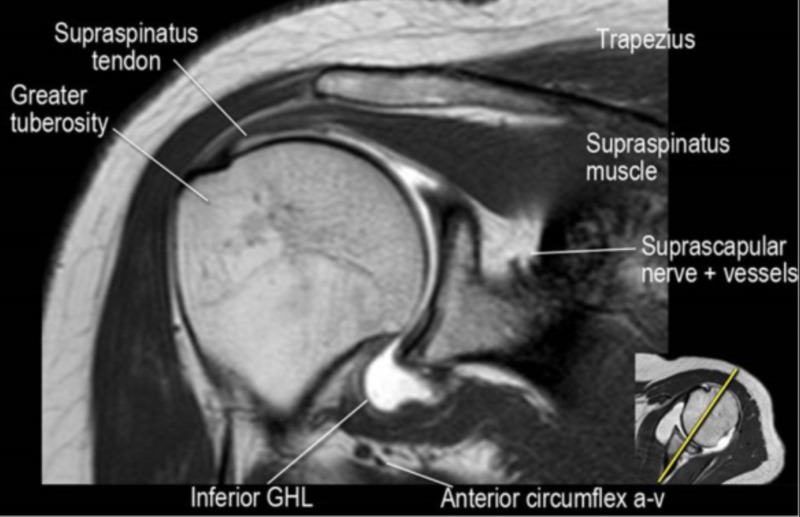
Normal shoulder MRI Magnetic resonance imaging (MRI) showing normal anatomy. GHL: glenohumeral ligament; a-v: artery/vein
